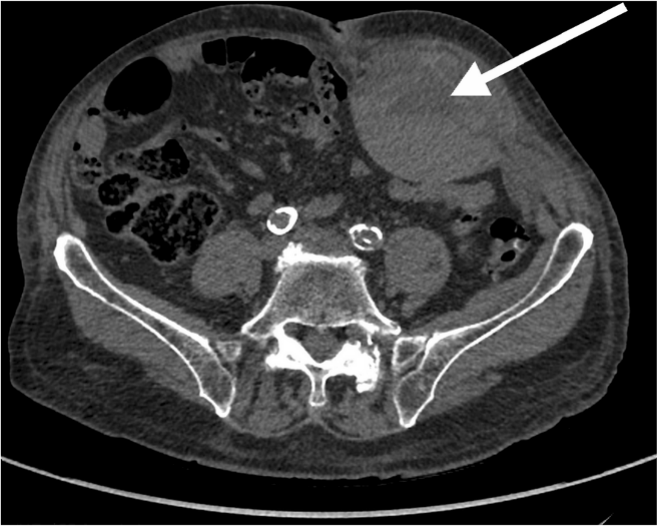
Abdominal CT scan. Hematoma of the rectus abdominis (White arrow).
